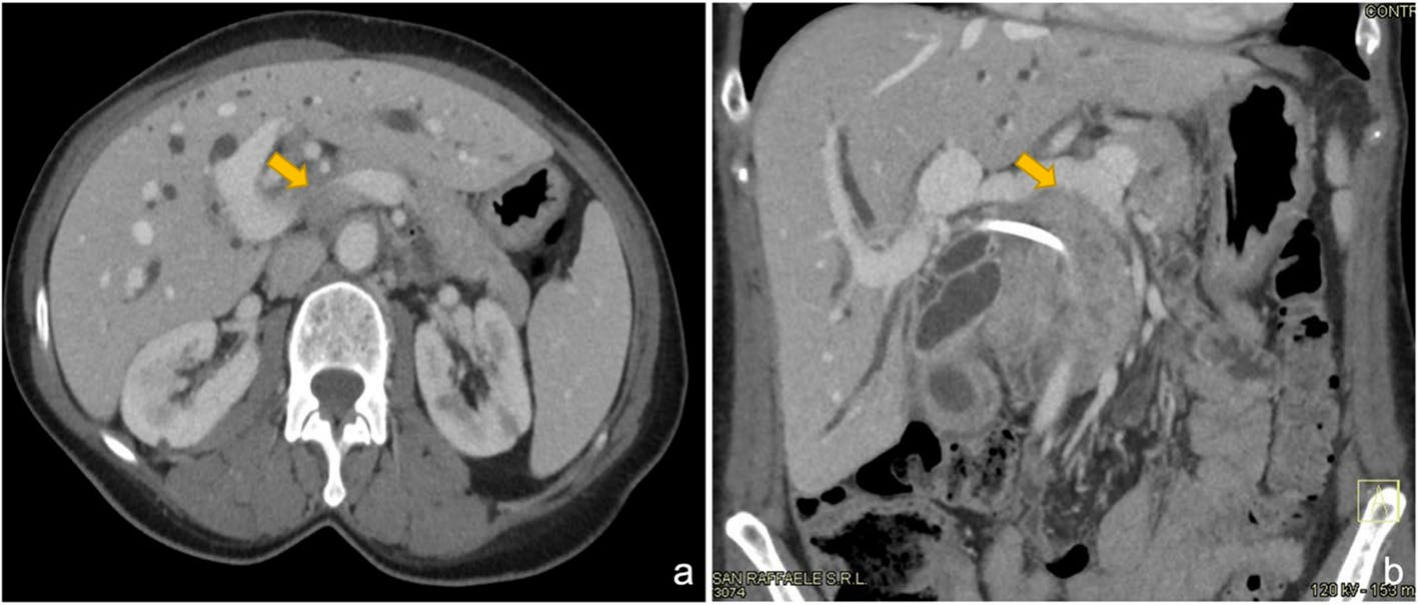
Axial (a) and coronal (b) preoperative CT sections showing narrowing of the portal trunk (yellow arrow) above spleno-mesenteric confluence
